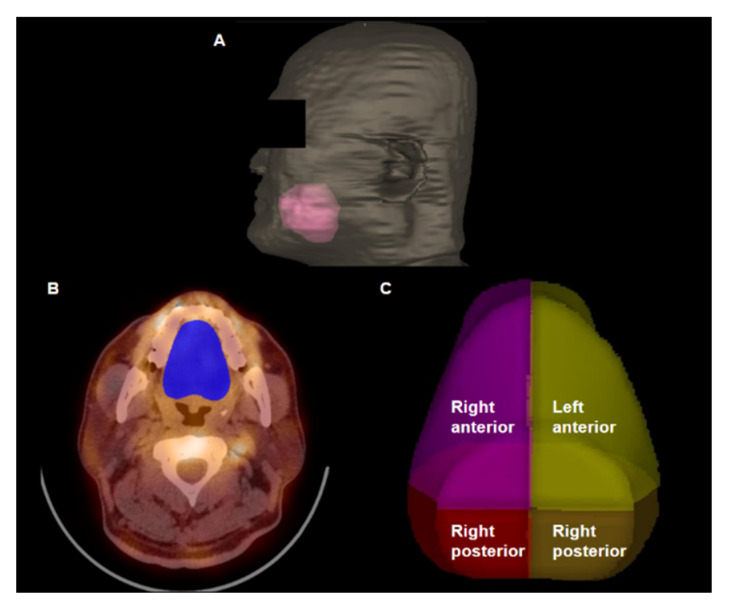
A volume rendering computed tomography image (A) and transaxial images (B,C) of volumes-of-interest of the tongue. The whole tongue area was delineated using a radiation treatment planning software (pink color on (A) and blue color on (B)). The whole tongue area was divided into four quadrant areas (C). Maximum standardized uptake value and total tongue uptake of the whole tongue and four quadrant areas were calculated using these volumes of interest.
